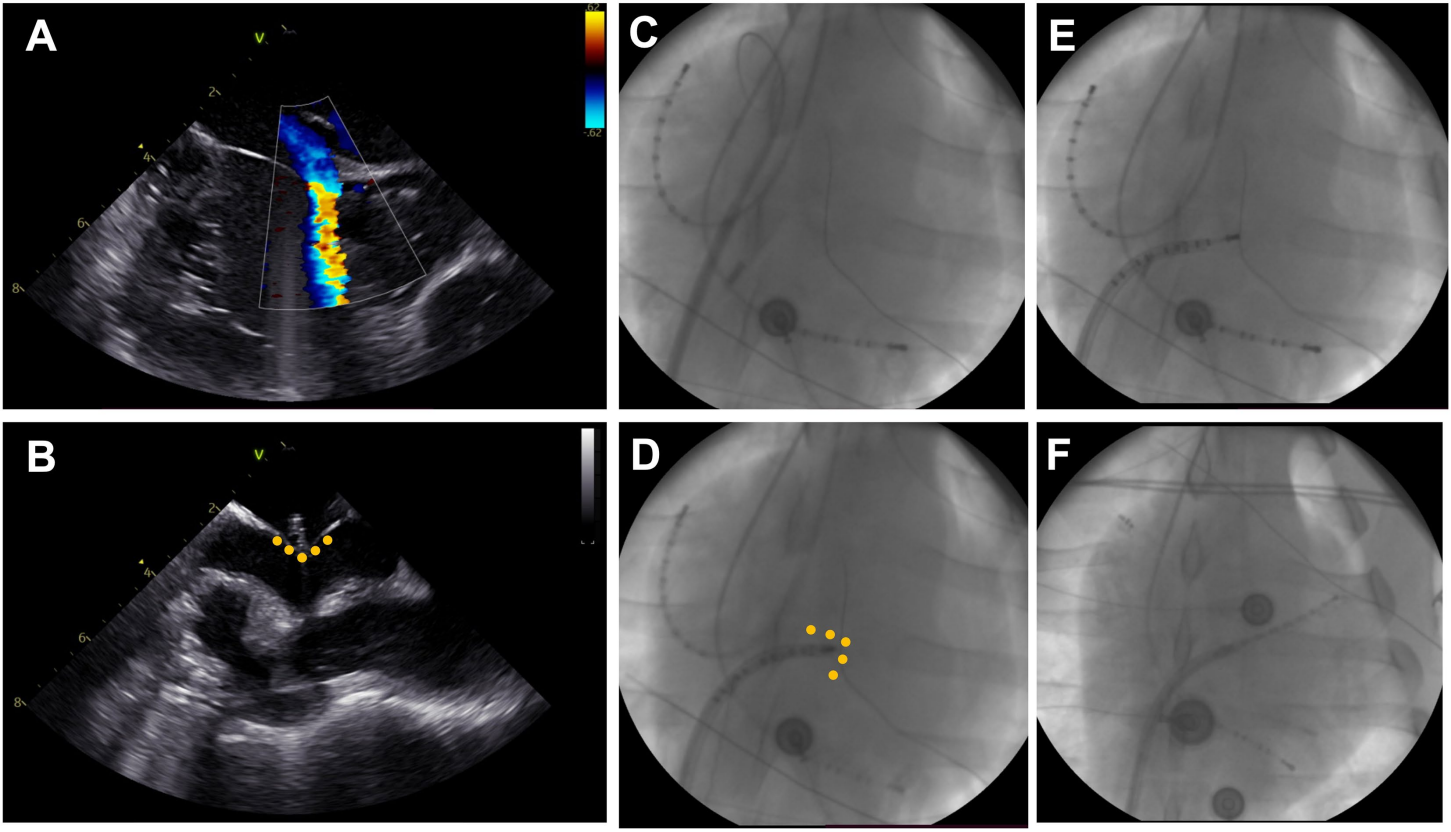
Transseptal puncture. (A) Visualization of a patent foramen ovale with right-to-left shunt on intracardiac echocardiography (ICE). (B) ICE-Visualization of blunt transseptal puncture through fossa ovalis showing tenting (yellow dots) and narrow left atrium. (C) Fluoroscopy image in anterior–posterior projection with decapolar catheter in high right atrium (left top) and coronary sinus (bottom right). The steerable sheath is advanced into the superior vena cava over the wire. (D) The sheath is retracted to the fossa ovalis and a diagnostic catheter is advanced. (E) Blunt passage of the fossa ovalis using the diagnostic catheter. (F) Positioning of the diagnostic catheter in the left atrial appendage and advancement of the steerable sheath into the left atrium.
